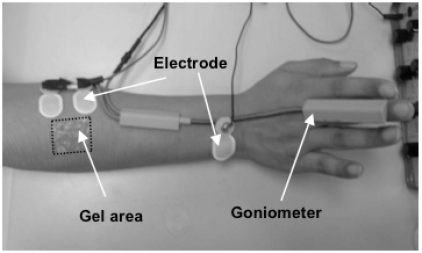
The experimental setup for the tests of the effects of the ultrasound gel on the SEMG collection. The gel areas tested were 0, 1, 6, 10 and 24 cm^2^ respectively.
